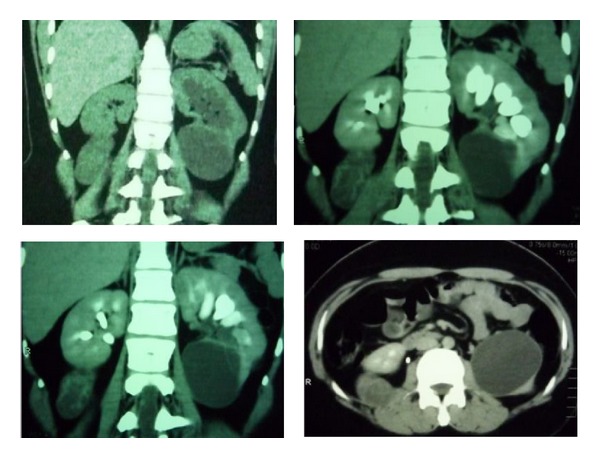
CT images demonstrate a retroperitoneal mass on the right side with heterogenous contrast enhancement and large cortical cyst in the lower pole of the left kidney with thin peripheral contrast enhancement without any other organ involvement.
